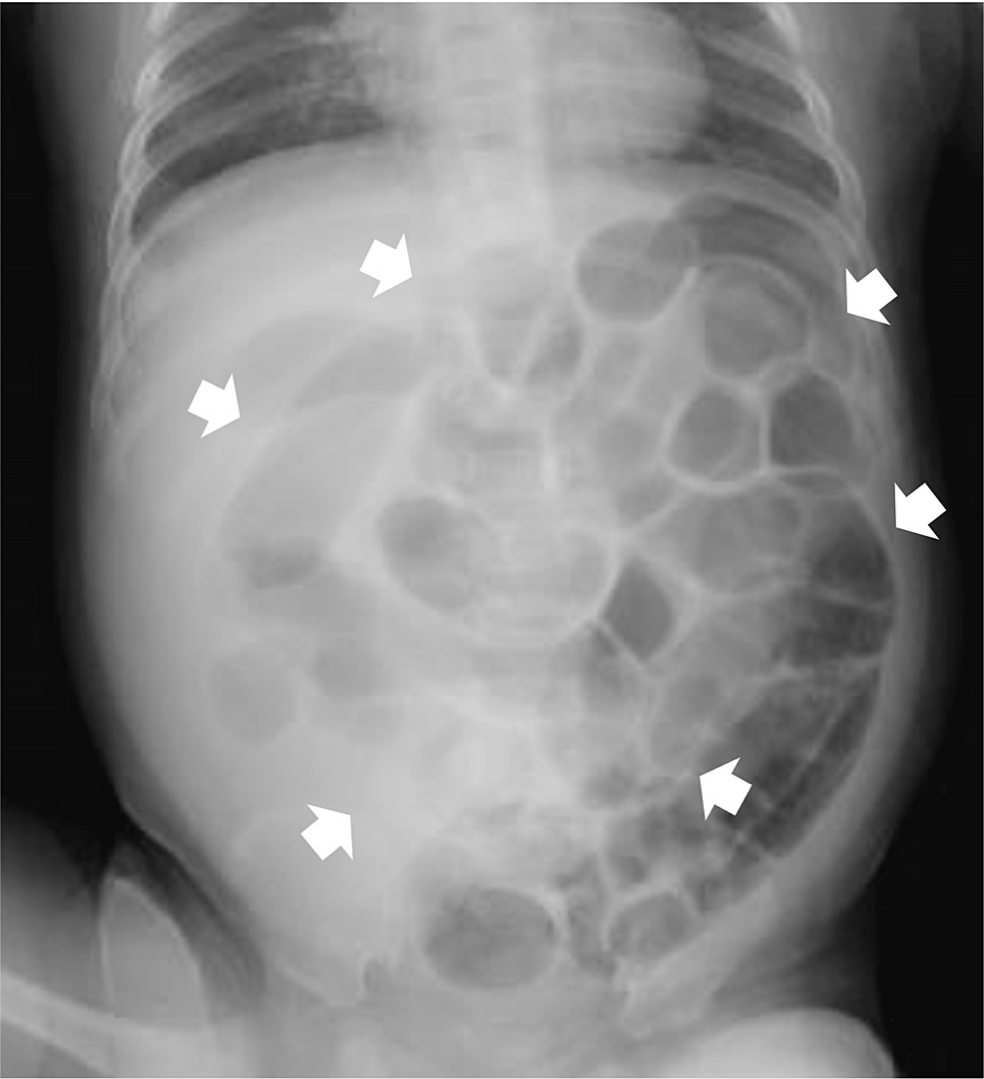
Abdominal radiograph at the spine position showing an extensive dilated small intestine
